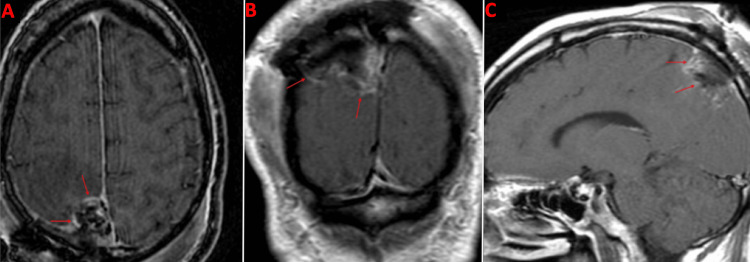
Postoperative MRI brain post contrast in the axial (A), coronal (B), and sagittal (C) planes Red arrows: Peripheral enhancement is seen at the surgical cavity which most likely represents Surgicel
